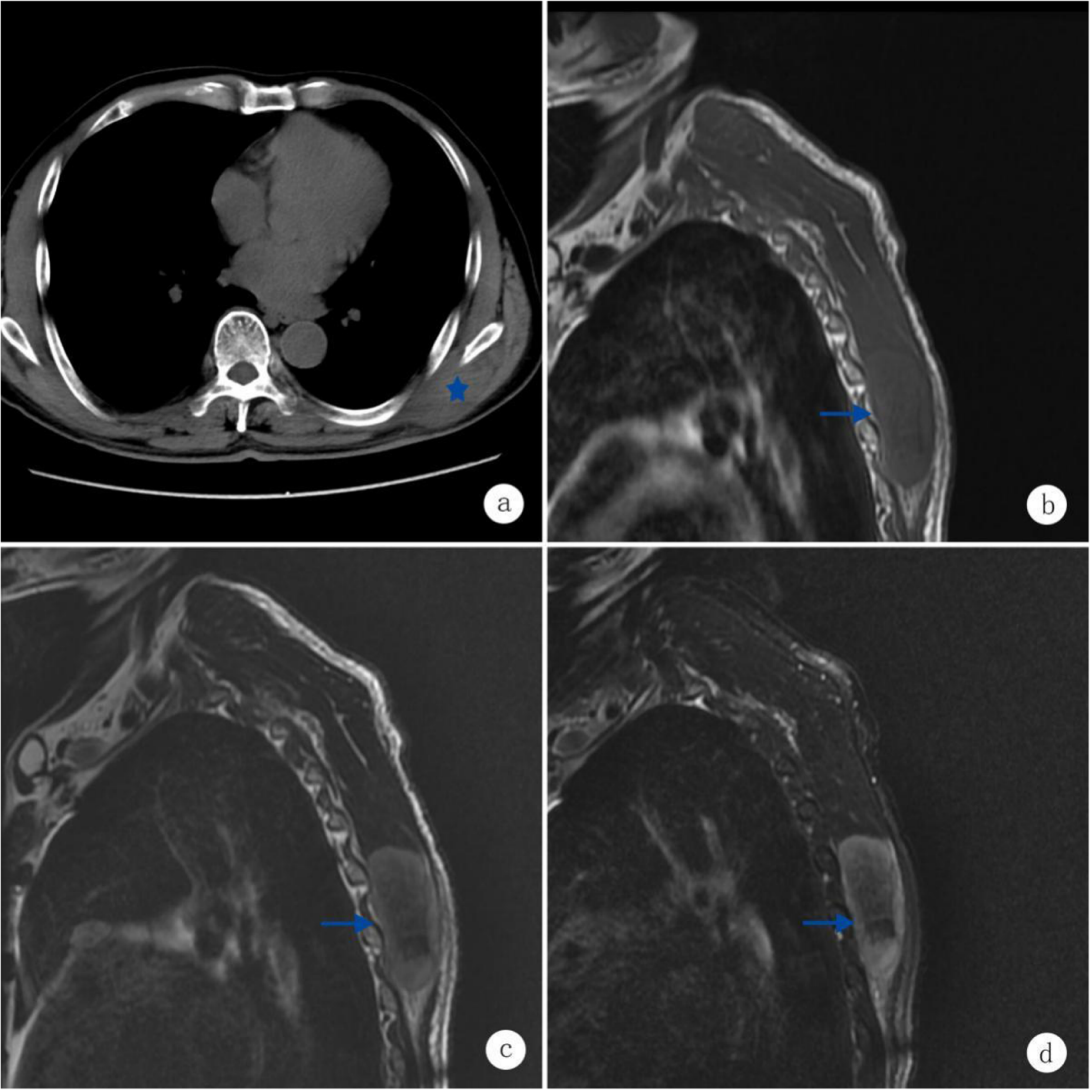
Imaging examination. a CT revealed an irregularly shaped soft tissue mass 7.0 cm × 5.4 cm × 2.7 cm in size in the left thoracic and dorsal muscle space, and the mass showed a clear boundary and uniform density. b MRI confirmed the presence of an irregularly shaped soft tissue mass in the left thoracic and dorsal muscle space with long T1. c T2 signals, as well as a uniform low signal on T1WI. d a high signal with fat suppression on T2WI. The blue five-pointed star and arrow indicate the mass
